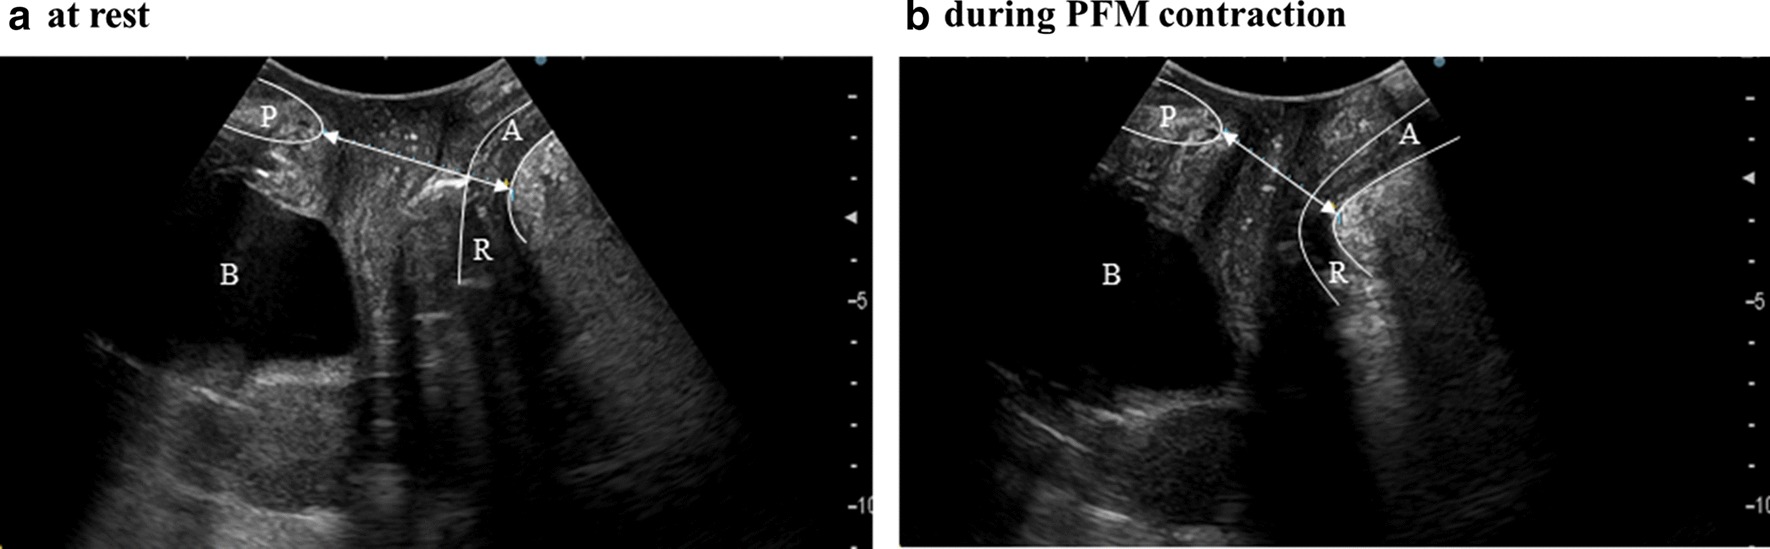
Transperineal mid-sagittal plane ultrasound image in healthy women: At rest (a), during pelvic floor muscle contraction (b). Urogenital hiatus diameter was measured as the distance between the anorectal junction and the inferior border of the pubic symphysis (white double headed arrow). The levator ani muscle was determined based on the hyperechogenic region posterior to the anorectal junction. The correct pelvic floor muscle contraction indicated the cranial ventral displacement of the levator ani muscle, and urogenital hiatus diameter was shortened. *A* anus, *B* bladder, *P* pubic symphysis, *R* rectum
